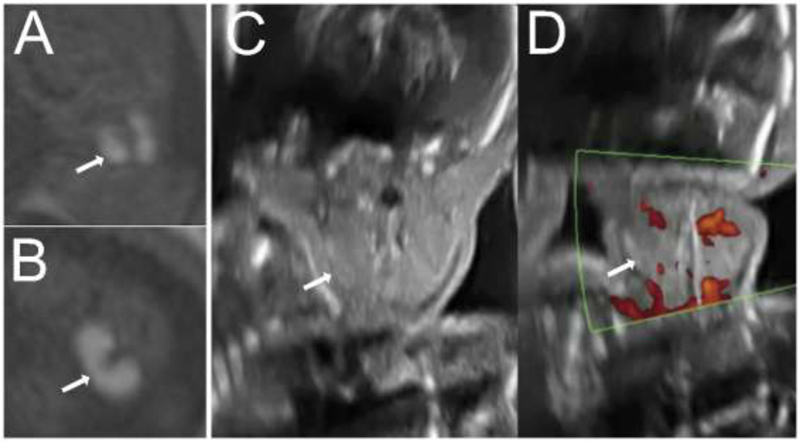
Fetus 2 at 22 weeks. Magnetic resonance imaging T1-weighted sequences of the neck showing the hyperintense signal of the thyroid gland (A, coronal; and B, axial), ultrasound images of the thyroid gland with and without color Doppler (C and D).
